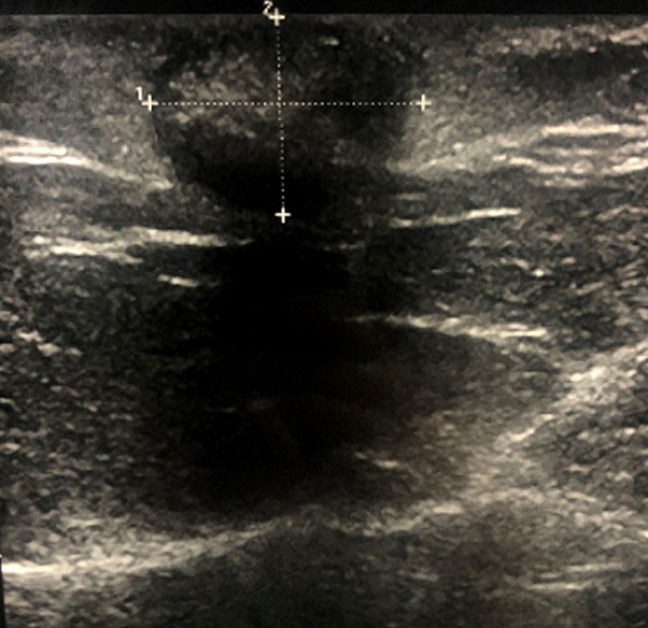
ultrasound showing very limited and homogeneous subcutaneous calcification
